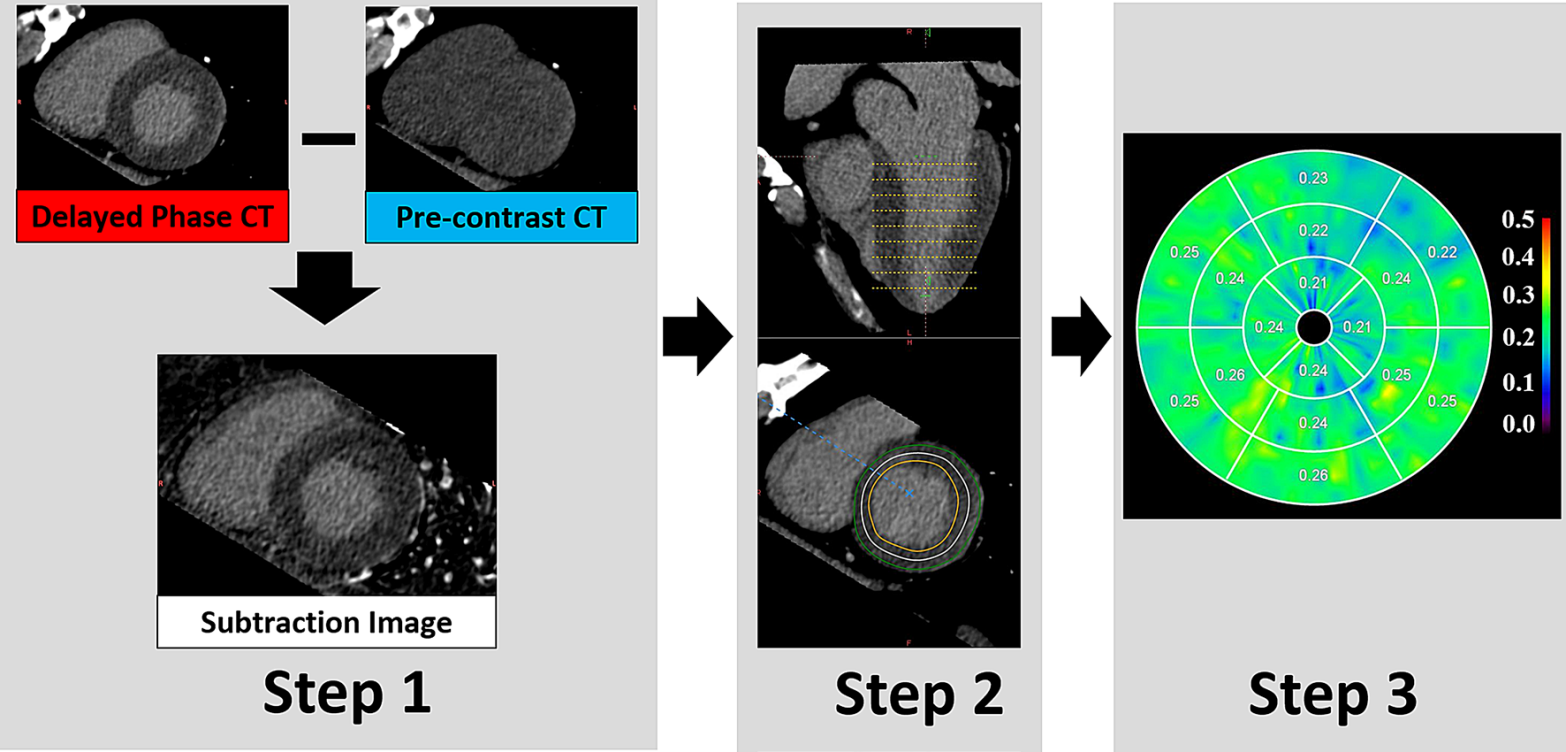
The process of calculating myocardial extracellular volume (ECV). Step 1: Generation of subtraction image. Subtraction images are generated from pre-contrast and delayed-phase CT by using an automatic three-dimensional non-rigid image registration. Step 2: Determination of left ventricular (LV) axis and segmentation of LV myocardium (tracing of endocardial and epicardial border). This step is also automated in the software with function of manually correction. A region of interest is placed in the LV cavity on the subtraction image to obtain ΔHUb. Step 3: Generation of ECV polar map. Entering the hematocrit value gives the ECV values for each segment in a polar map. In the polar map, ECV values are displayed as fractions.
